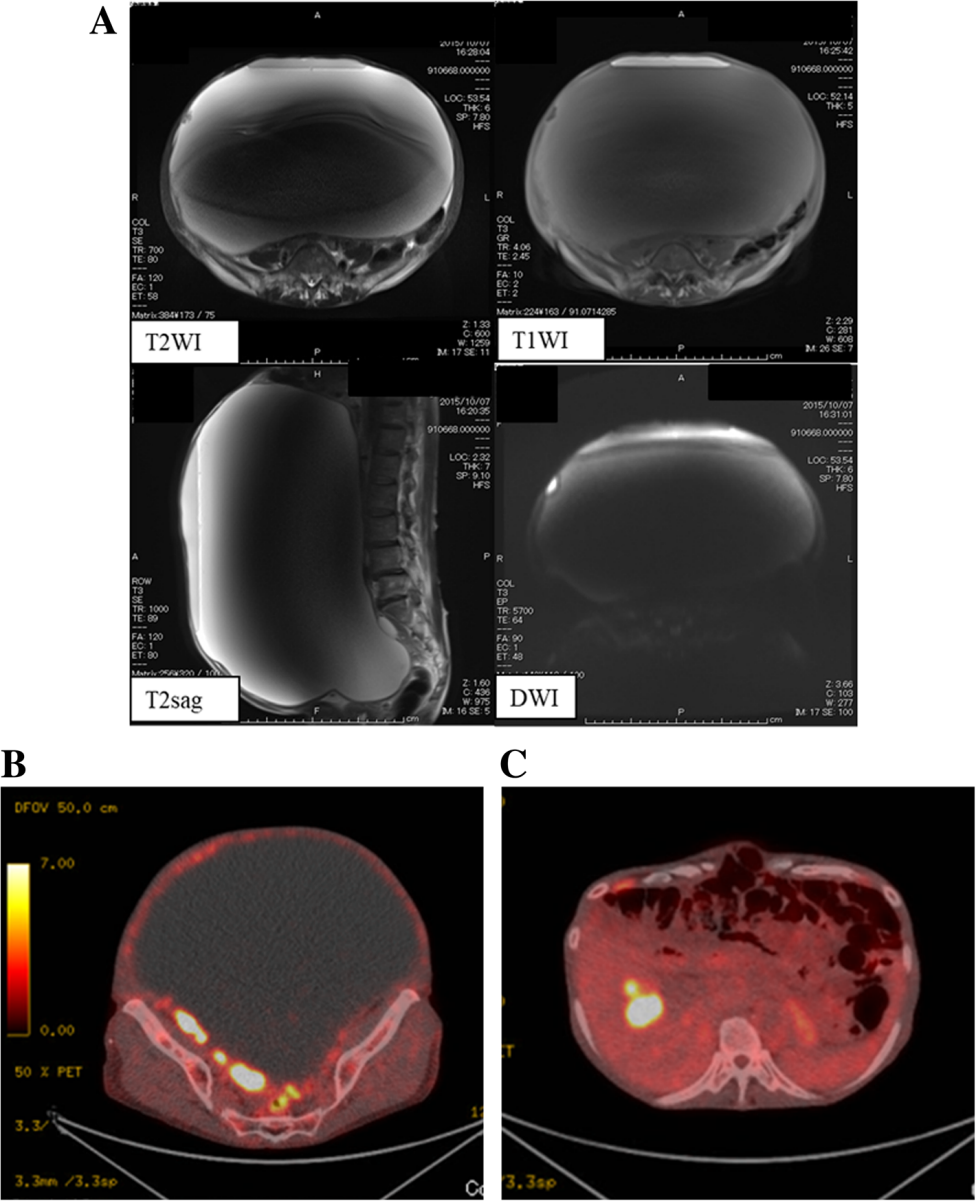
a. MRI findings: Horizontal T2-weighted and T1-weighted MR imaging demonstrated a water component which showed a drop in signal intensity with fat. b. FDG-PET showed FDG uptake in the pelvic tumor (SUVmax = 22.9). c. The hepatic nodule also showed FDG uptake (SUVmax = 13.7), which was suggestive of metastases
